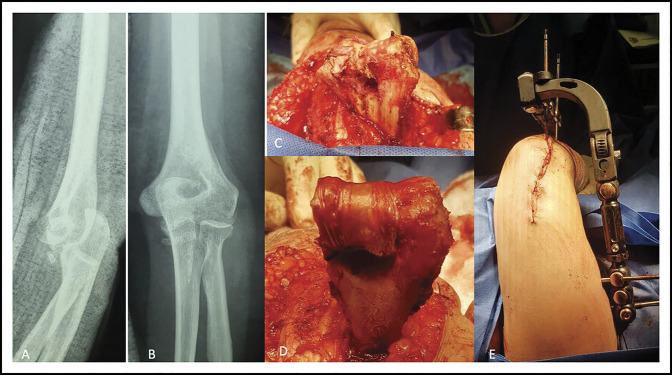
A and B, Presurgical AP and lateral radiographic view of our patient. C, Trans-surgical images showing abundant chondral lesion. D, Placement of interposition arthroplasty. E, Articulated external fixation with cutaneous closure.
